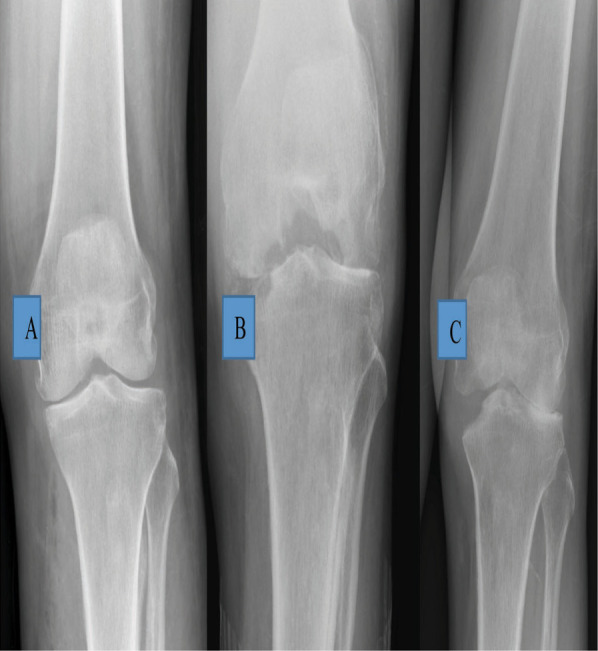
Radiograph (taken elsewhere) - AP view of the left knee just before the last intra-articular injection was given, showing that the medial joint space is narrowed, but the femoral and tibial condyles are not destroyed, Radiographs AP and lateral views of the same knee taken a month later, on admission, show marked destruction of femoral and tibial condyles. Such rapid destruction of bone is commonly seen due to pyogenic infection. Posterior subluxation of tibia seen in Lateral view, AP radiographs of the both knees 9 months post-arthrotomy and antibiotic therapy, shows some reformation of the medial femoral and tibial condyles.
